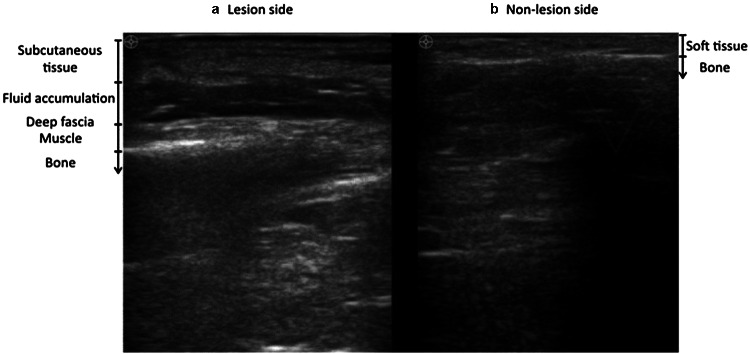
Ultrasonography shows fluid accumulation in the deep fascial layer. a The Lesion side had clinically suspected NF. b Non-lesion side had a normal skin appearance. Source: Lin et al. with permission of the author
